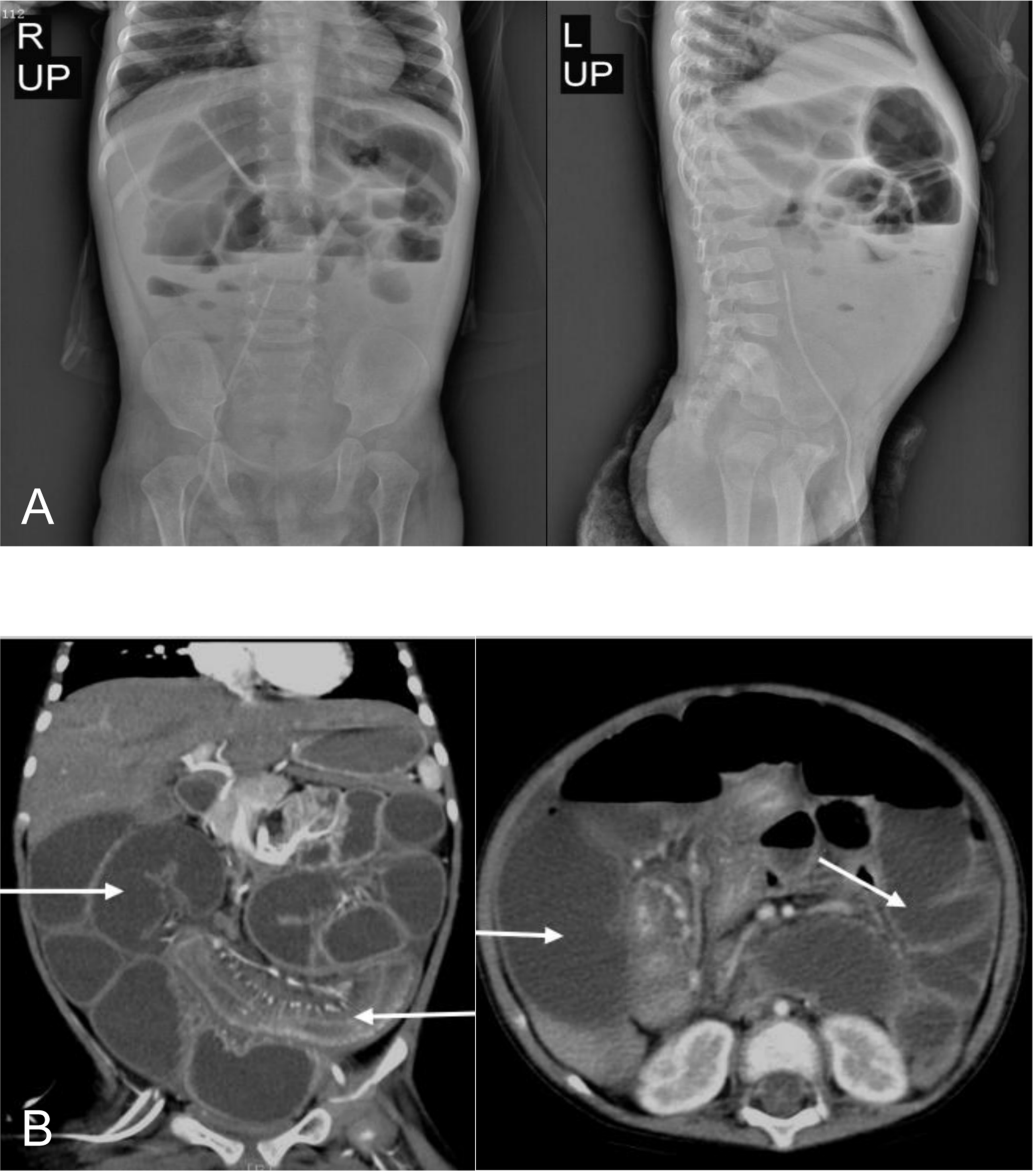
Bowel dilation, bowel wall thickening, and stenosis in the patient. (A) Abdominal radiograph showed bowel dilation with a gas−liquid level. (B) CT scan showed bowel dilation, thickening of the bowel wall, and small bowel stenosis.
